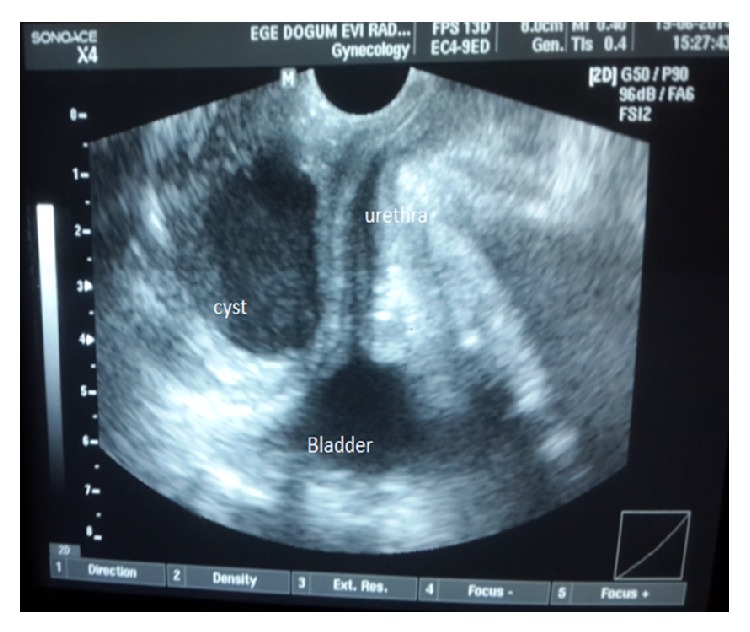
Perineal ultrasonography revealed no relationship between the cyst and the lower urinary tract.
